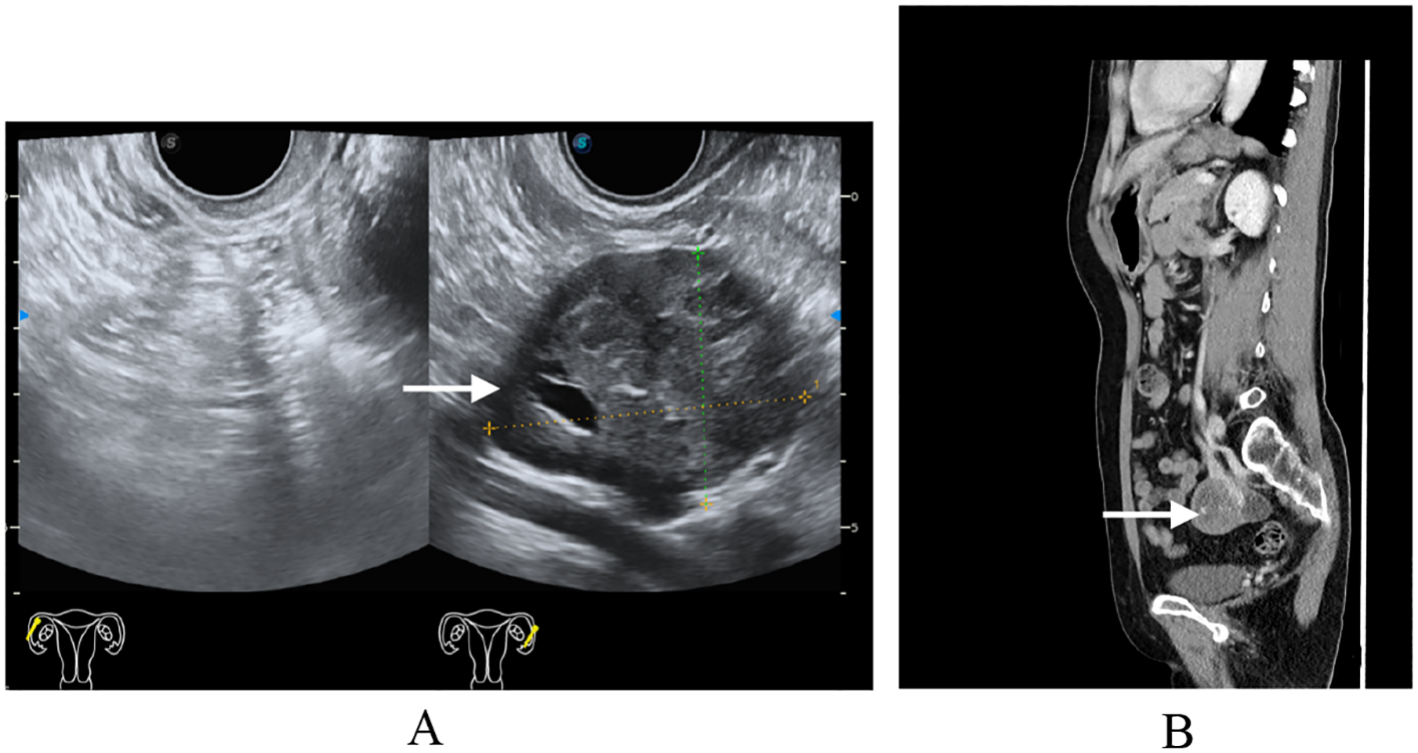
Preoperative imaging findings. (A) Transvaginal ultrasound: single-chamber anechoic cystic lesion, 5 cm in diameter, arising from the left adnexa; wall thin and smooth, no internal septa or papillary projections, color Doppler shows absent vascularity. (B) Computed tomography same-level non-contrast CT reveals a well-circumscribed, oval low-density cystic mass in the left adnexal region, homogeneous attenuation, no enhancement, adjacent structures mildly displaced without mass effect.
